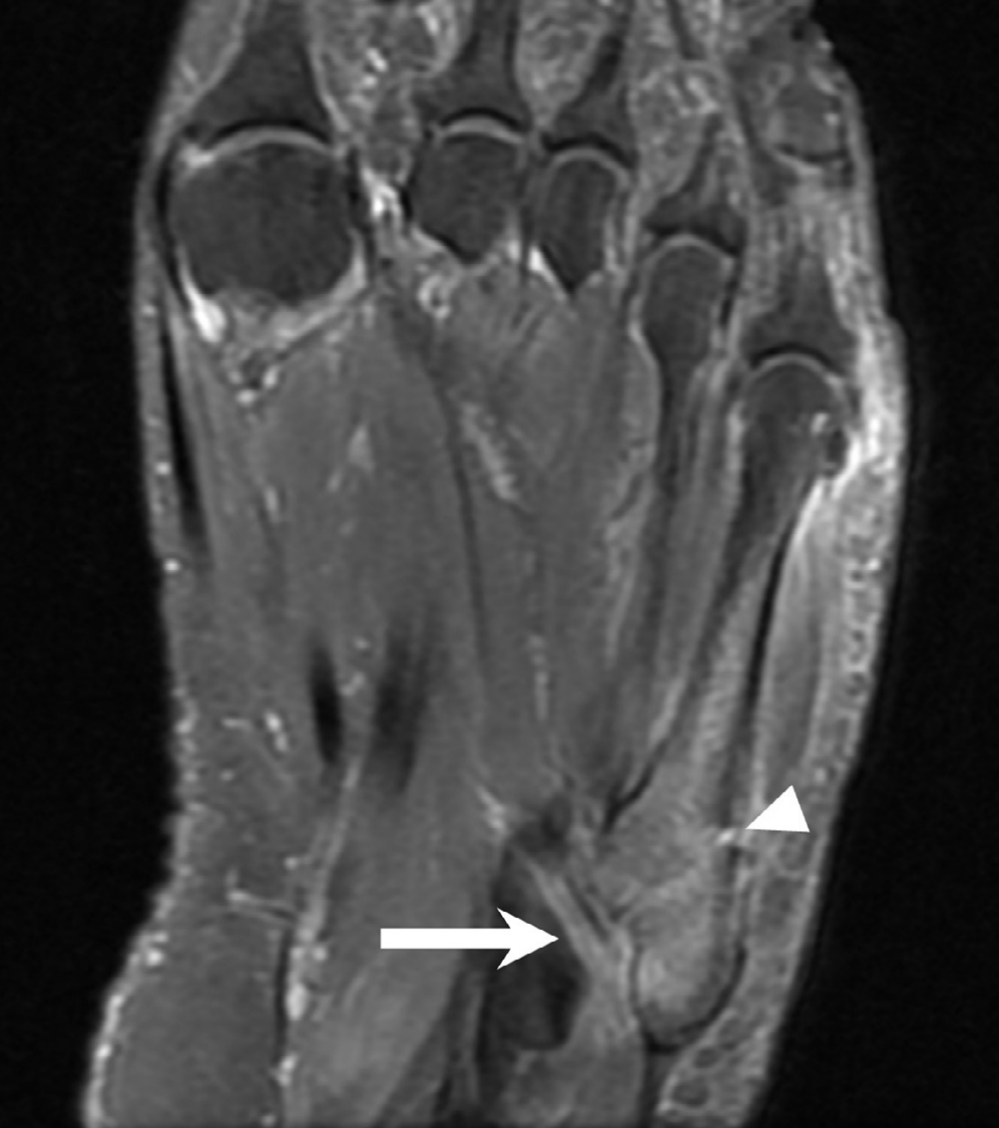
Proton density fat sat MRI sequence of the right foot shows a fracture at the base of the fifth metatarsal (arrowhead) with associated bone marrow edema and peripheral soft tissue edema. Due to known tendon tear and retraction, the PLT is not seen and there is fluid signal in its expected location (arrow).
